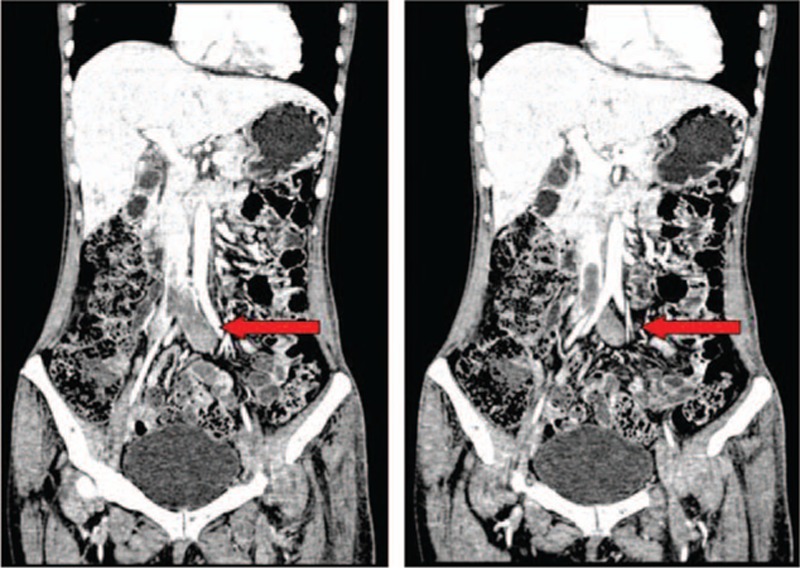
Abdominal–pelvic-enhanced computed tomography scan showing the thrombosis from inferior vena cava to the left tibial veins.
